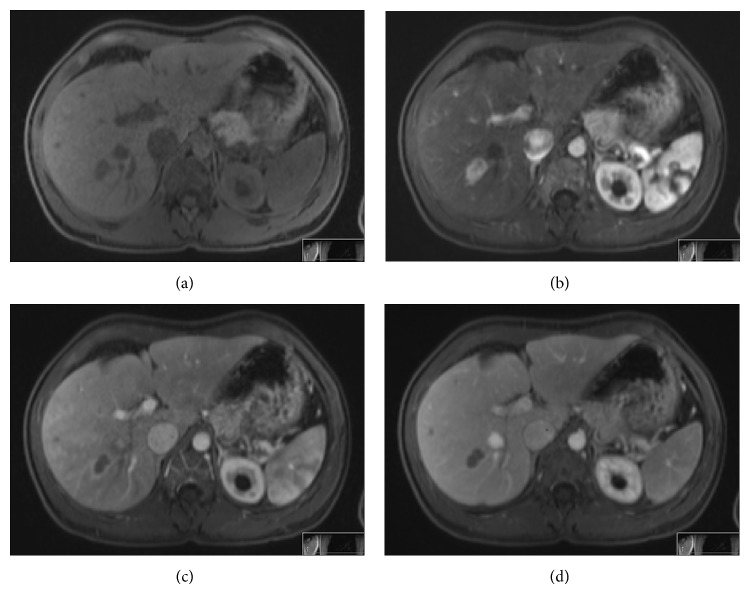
MRI axial T1-weighted images of the liver lesion before and after intravenous contrast medium administration. (a) Preintravenous contrast medium administration; focal lesion in segment VII of the liver and (b) arterial phase; early enhancement following contrast administration, and (c, d) venous and equilibrium phase, respectively, early washout.
